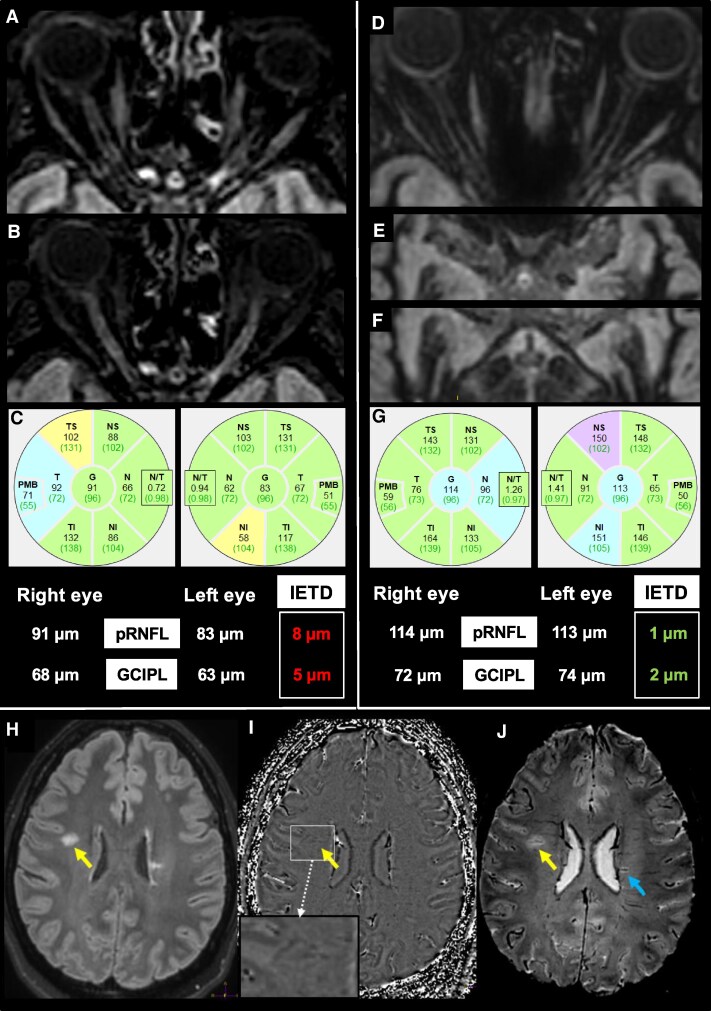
Brain/optic nerves MRI and OCT finding in patients with radiologically isolated syndrome. (A, B) A patient with RIS presented with a left canalicular optic nerve lesion on 3D-DIR (A) and 3D FLAIR (B) axial reconstructions. OCT also showed a decreased pRNFL and GCIPL on the left eye with pRNFL IETD of 8 µm and GCIPL-IETD of 5 µm, suggesting the presence of a left optic nerve lesion (C). (D–G) A patient with RIS presented without any lesion on the anterior visual pathways (optic nerves [D], Chiasma [E], optic tracts [F]) on axial 3D-DIR reconstruction. OCT showed comparable pRNFL and GCIPL with pRNFL-IETD and GCIPL-IETD (G) in normal ranges, confirming the absence of optic nerve lesion. (H–J) A patient with RIS presented with 3D-FLAIR hypersignals (H) suggesting demyelinating with a PRL on phase imaging (I) and CVS on SWI reconstruction (J). The yellow arrow is pointing a T2 lesion with PRL and CVS. The blue arrow is pointing another T2 lesion with CVS.
